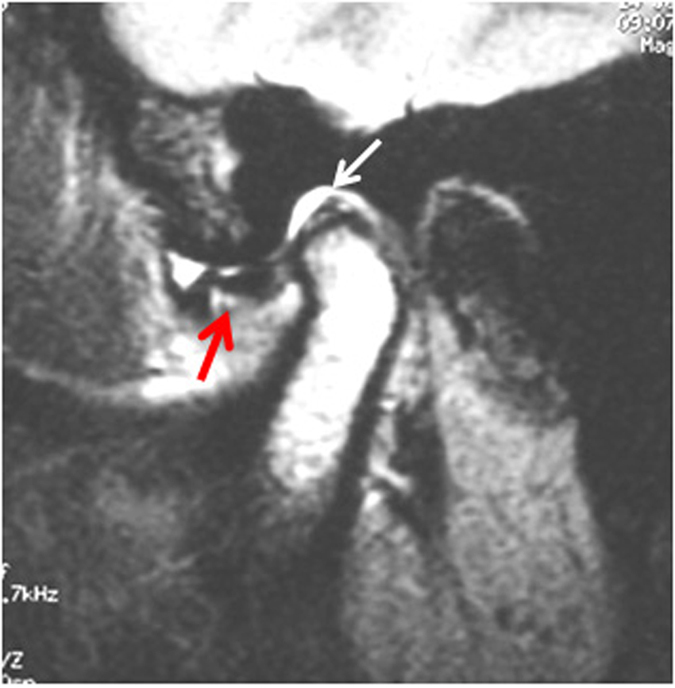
MRI showed acute phase of ATDD, disc displaced in front of the condyle (red arrow) with normal shape and length. The posterior band was disrupted and elongated with or without effusion (white arrow).
